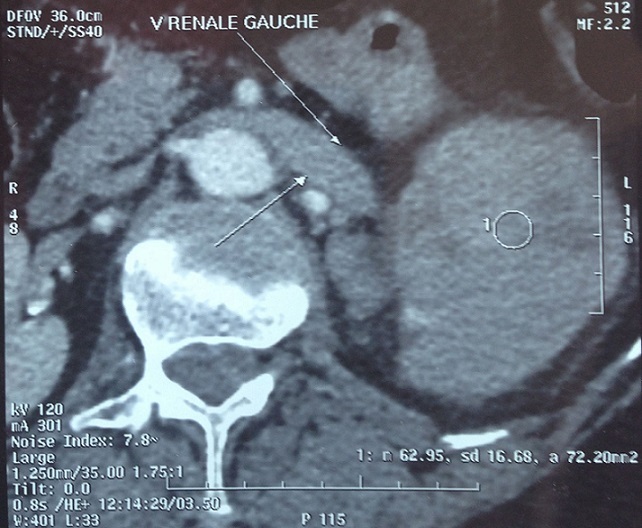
Abdominal and pelvic computed tomography scan showed that the process extends over the hilar area with invasion of the right renal vein, while the lower vena cava is permeable
